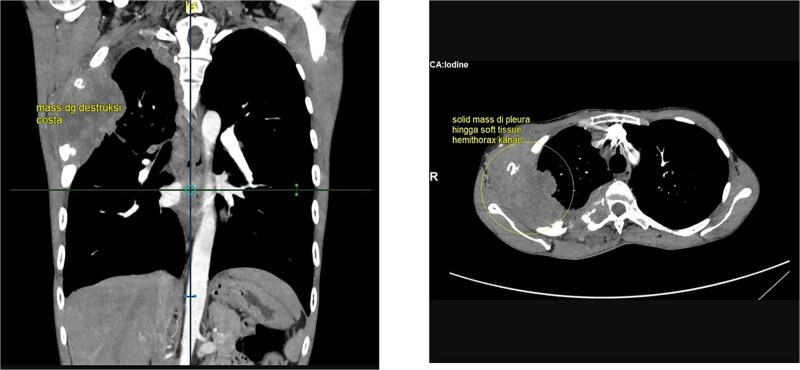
Contrast-enhanced CT scan of the thorax. Coronal and axial images reveal a large, heterogeneous mass occupying the right hemithorax, arising from the chest wall and extending into the pleura and adjacent soft tissues. The lesion demonstrates rib destruction, consistent with locally aggressive disease. Areas of internal low attenuation suggest necrosis. The mass abuts and partially invades the adjacent lung parenchyma, with irregular and spiculated margins.
